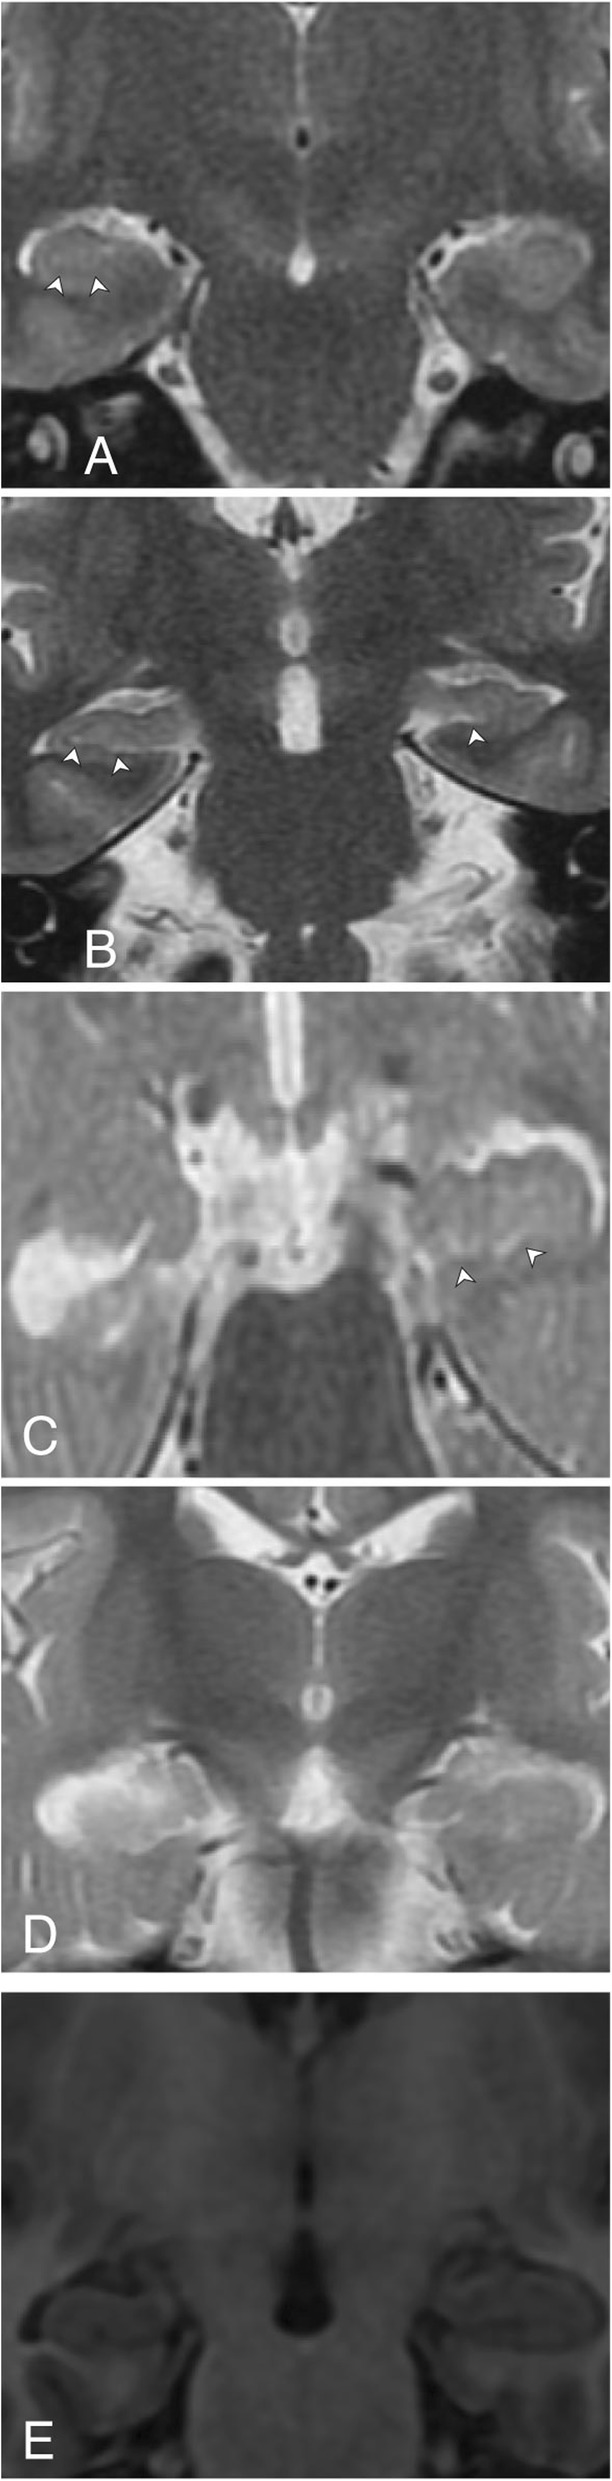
a–d High-resolution coronal T2-weighted images with magnification; e Coronal SPGR image with magnification. Arrowheads indicate hippocampal striation. a Left PLHS: hippocampal striation (arrowheads) seen better on the right side; not well made out on the left. Cochleae are seen symmetrical on both sides. b Left PLHS: hippocampal striation (arrowheads) seen better on the right side; seen only in medial aspect of the left hippocampus. Semicircular canals are seen symmetrical on both sides. c Right PLHS: hippocampal striation (arrowheads) seen on the left side; not on the right. The right hippocampus is also small, with increased signal intensity. d Bilateral PLHS: hippocampal striation not seen on either side; the right hippocampus is smaller in size. e Bilateral PLHS: small size of right hippocampus is also seen on coronal SPGR image
